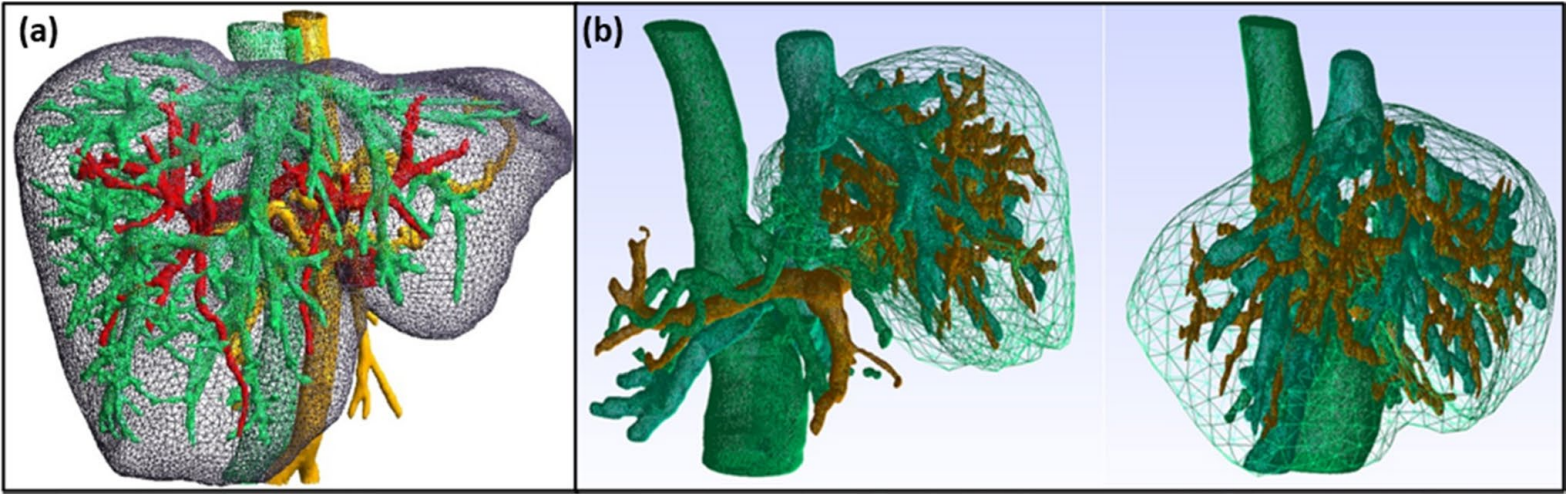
a The FE modeling of liver geometry and its vascularization with each cube representing a heterogeneous segment (Kugler 2018), with permission of HAL archives-ouvertes.fr, copyright 2018. b Example of liver and vascularization surface meshes obtained through segmentation of CT scan images from IRCAD with light mesh corresponding to the liver and other brown, blue-green, and green dense meshes corresponding to the vascularization (Michael Kugler et al. 2018a, b), with permission of Elsevier Ltd., copyright 2018.
